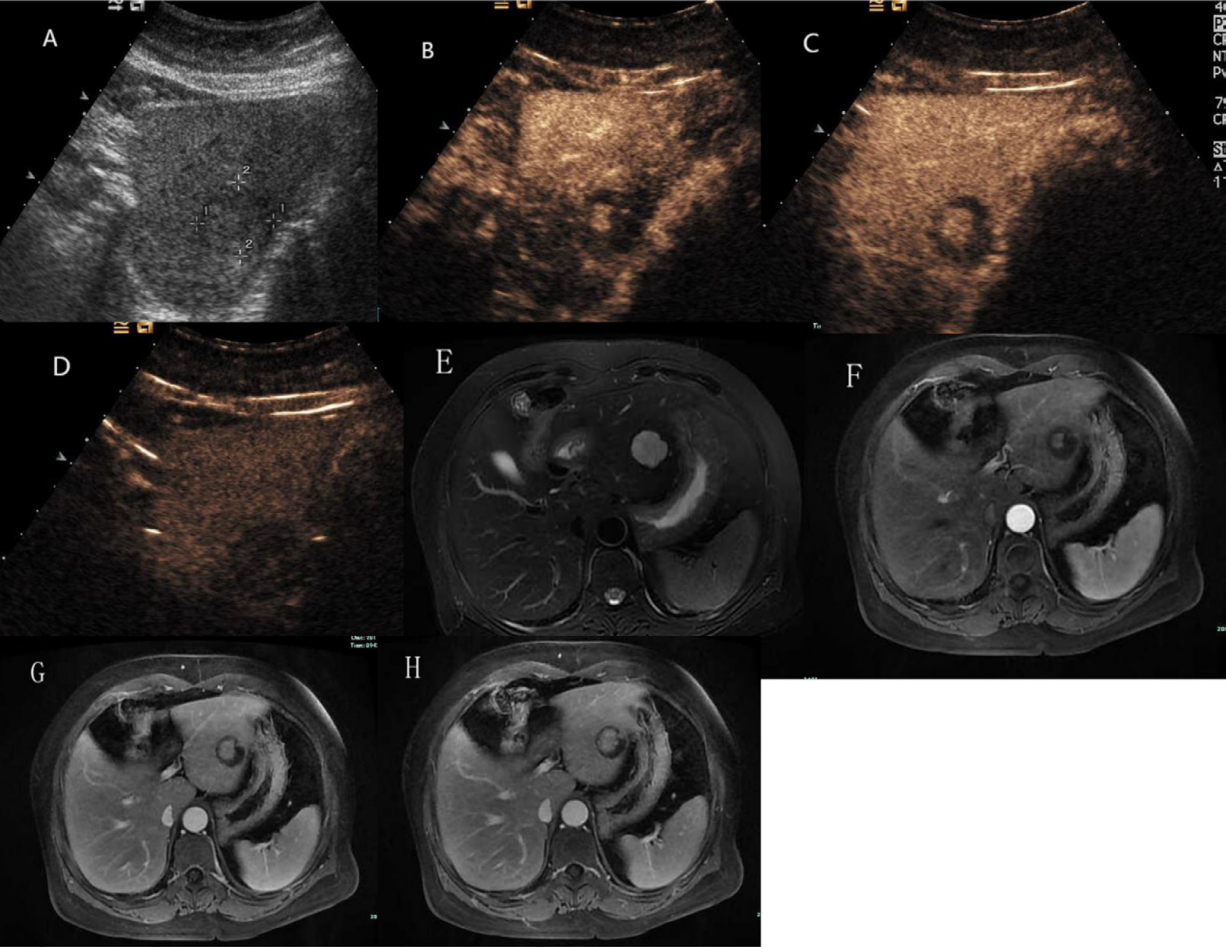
Hepatic hemangioma with centrifugal enhancement (A) Ultrasound revealed an isoechoic mass in the left liver. (B) and (C) CEUS showed a central enhancing foci in the arterial phase and followed by a centrifugal enhancement. (D) CEUS showed hypoechoic change in the delayed phase. (E) The lesion presented as markedly hyperintense on T2 weighted MR images. (F) Contrast enhanced MR images showed a central enhancing focus in the arterial phase. (G) and (H) The lesion showed centrifugal enhancement in the portal-venous phase and late phase.
